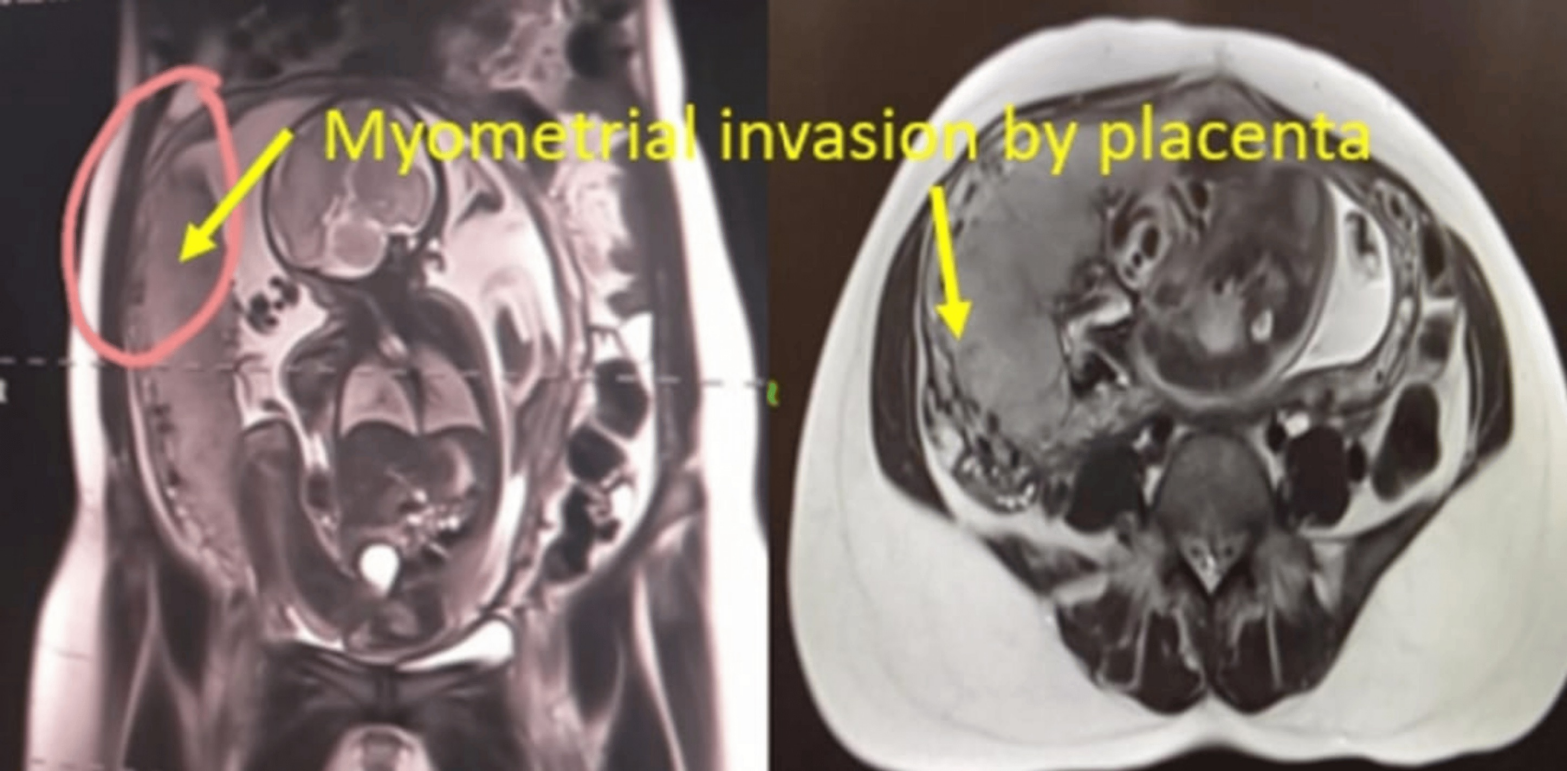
Placental adherence and invasion in the upper segment on MRI
